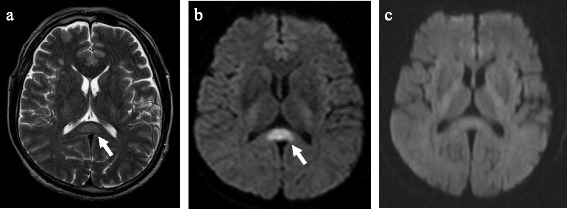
T2- and diffusion-weighted images. T2-weighted (a) and diffusion-weighted (b) brain magnetic resonance images on the 2^nd^ day showing high-intensity lesions in the splenium of the corpus callosum (*arrow*). The splenial lesions had completely disappeared on the 5^th^ day (c)
